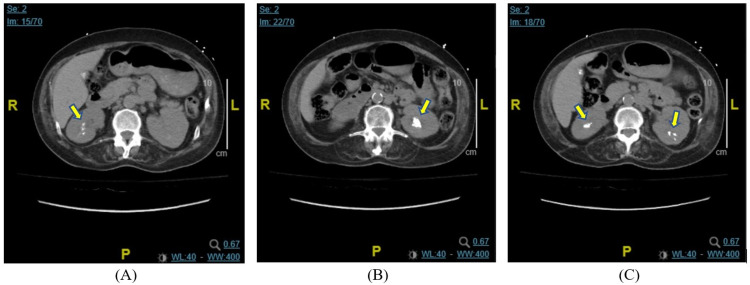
CT abdomen/pelvis without IV contrast in June 2021 Multiple renal stones are present in the bilateral lower poles of kidneys (arrows) CT: computed tomography; IV: intravenous
